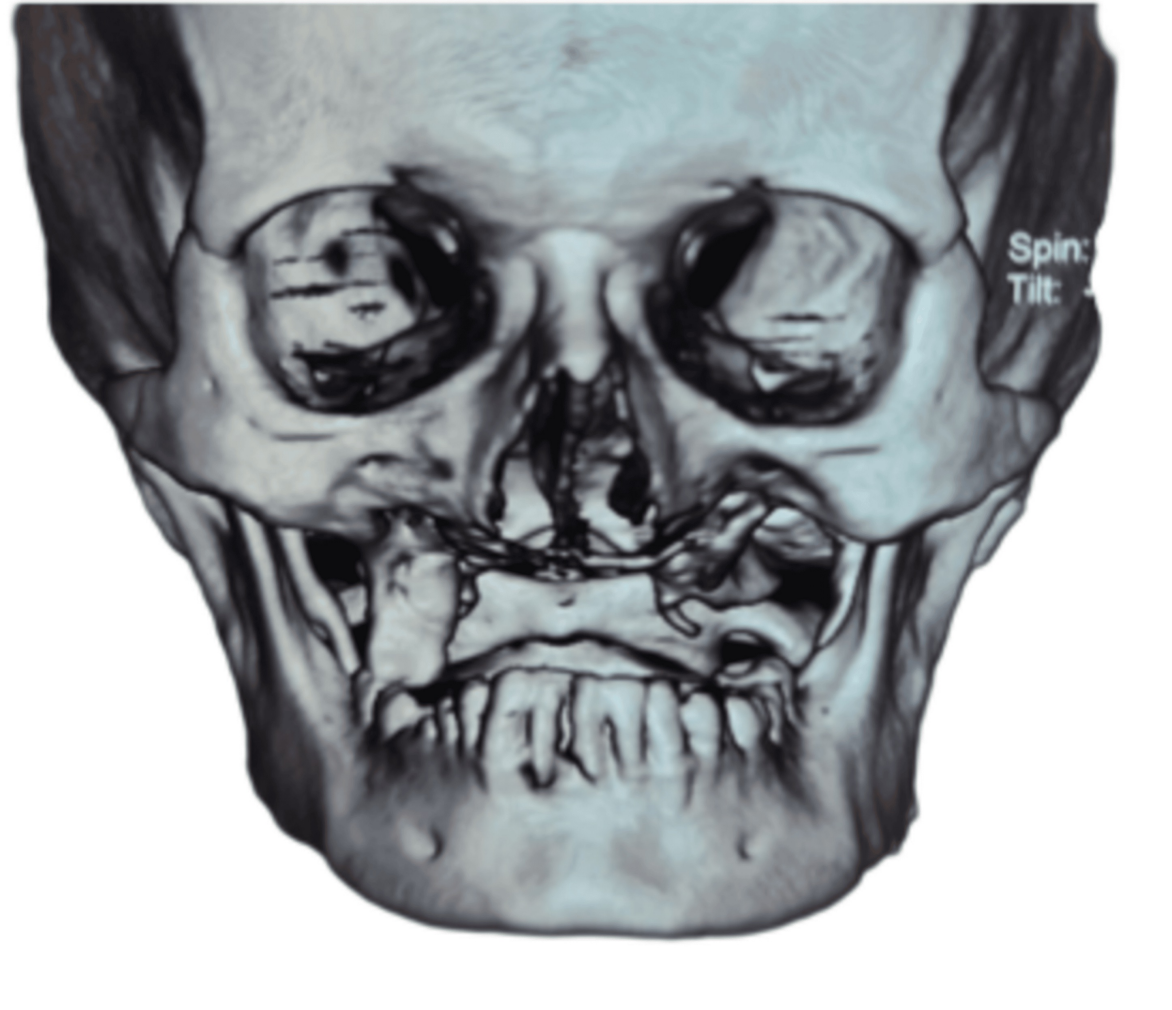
3D CT face for designing patient-specific implants
